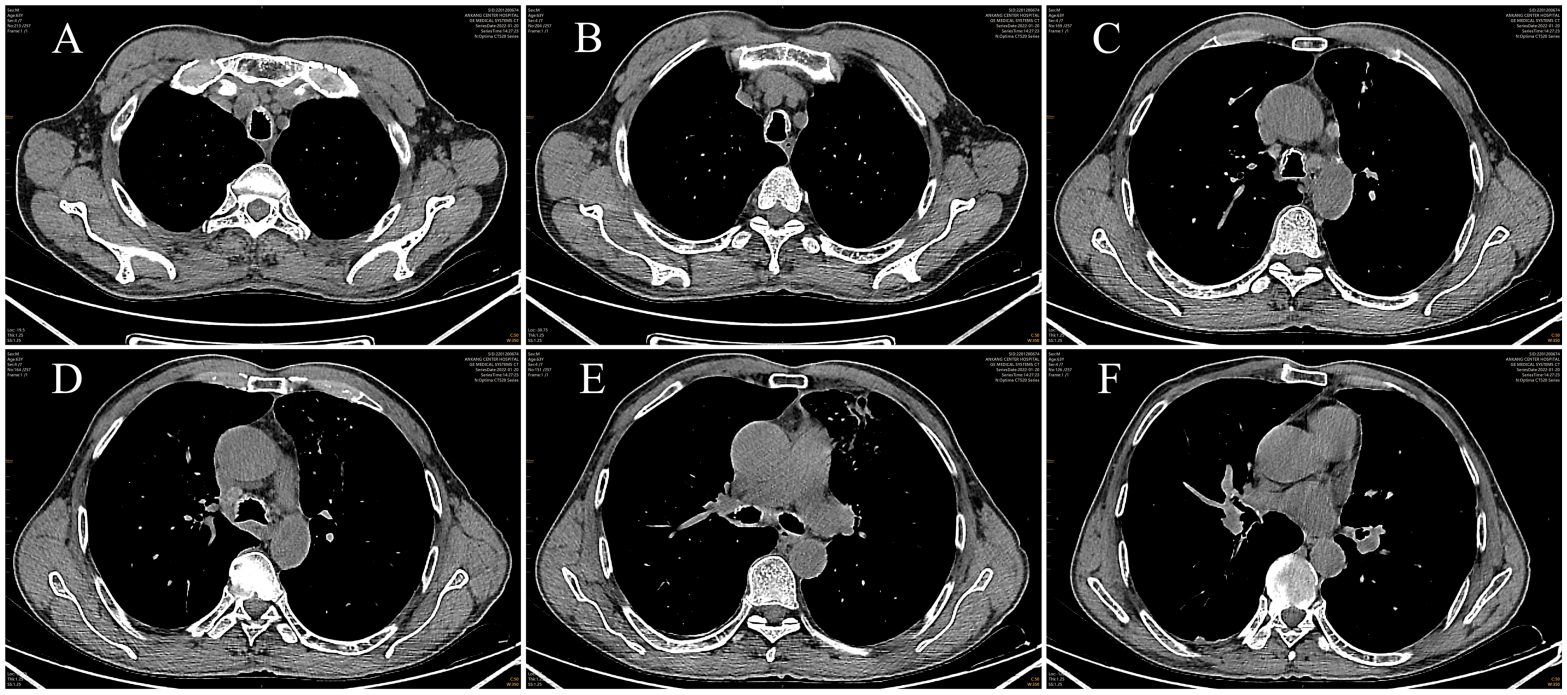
Images of mediastinal window from chest CT scan(a chest CT scan showed multiple calcified and non-calcified nodules protruding into the lumen of the trachea and main bronchi, with irregular luminal contours, sparing the posterior tracheal wall (membranous portion). ((A, B) Level Above the Aortic Arch, (C, D) Level at the Lower End of the Trachea, (E) Level of the Tracheal Bifurcation, (F) Level of the Right Middle Lobe Bronchus)).
